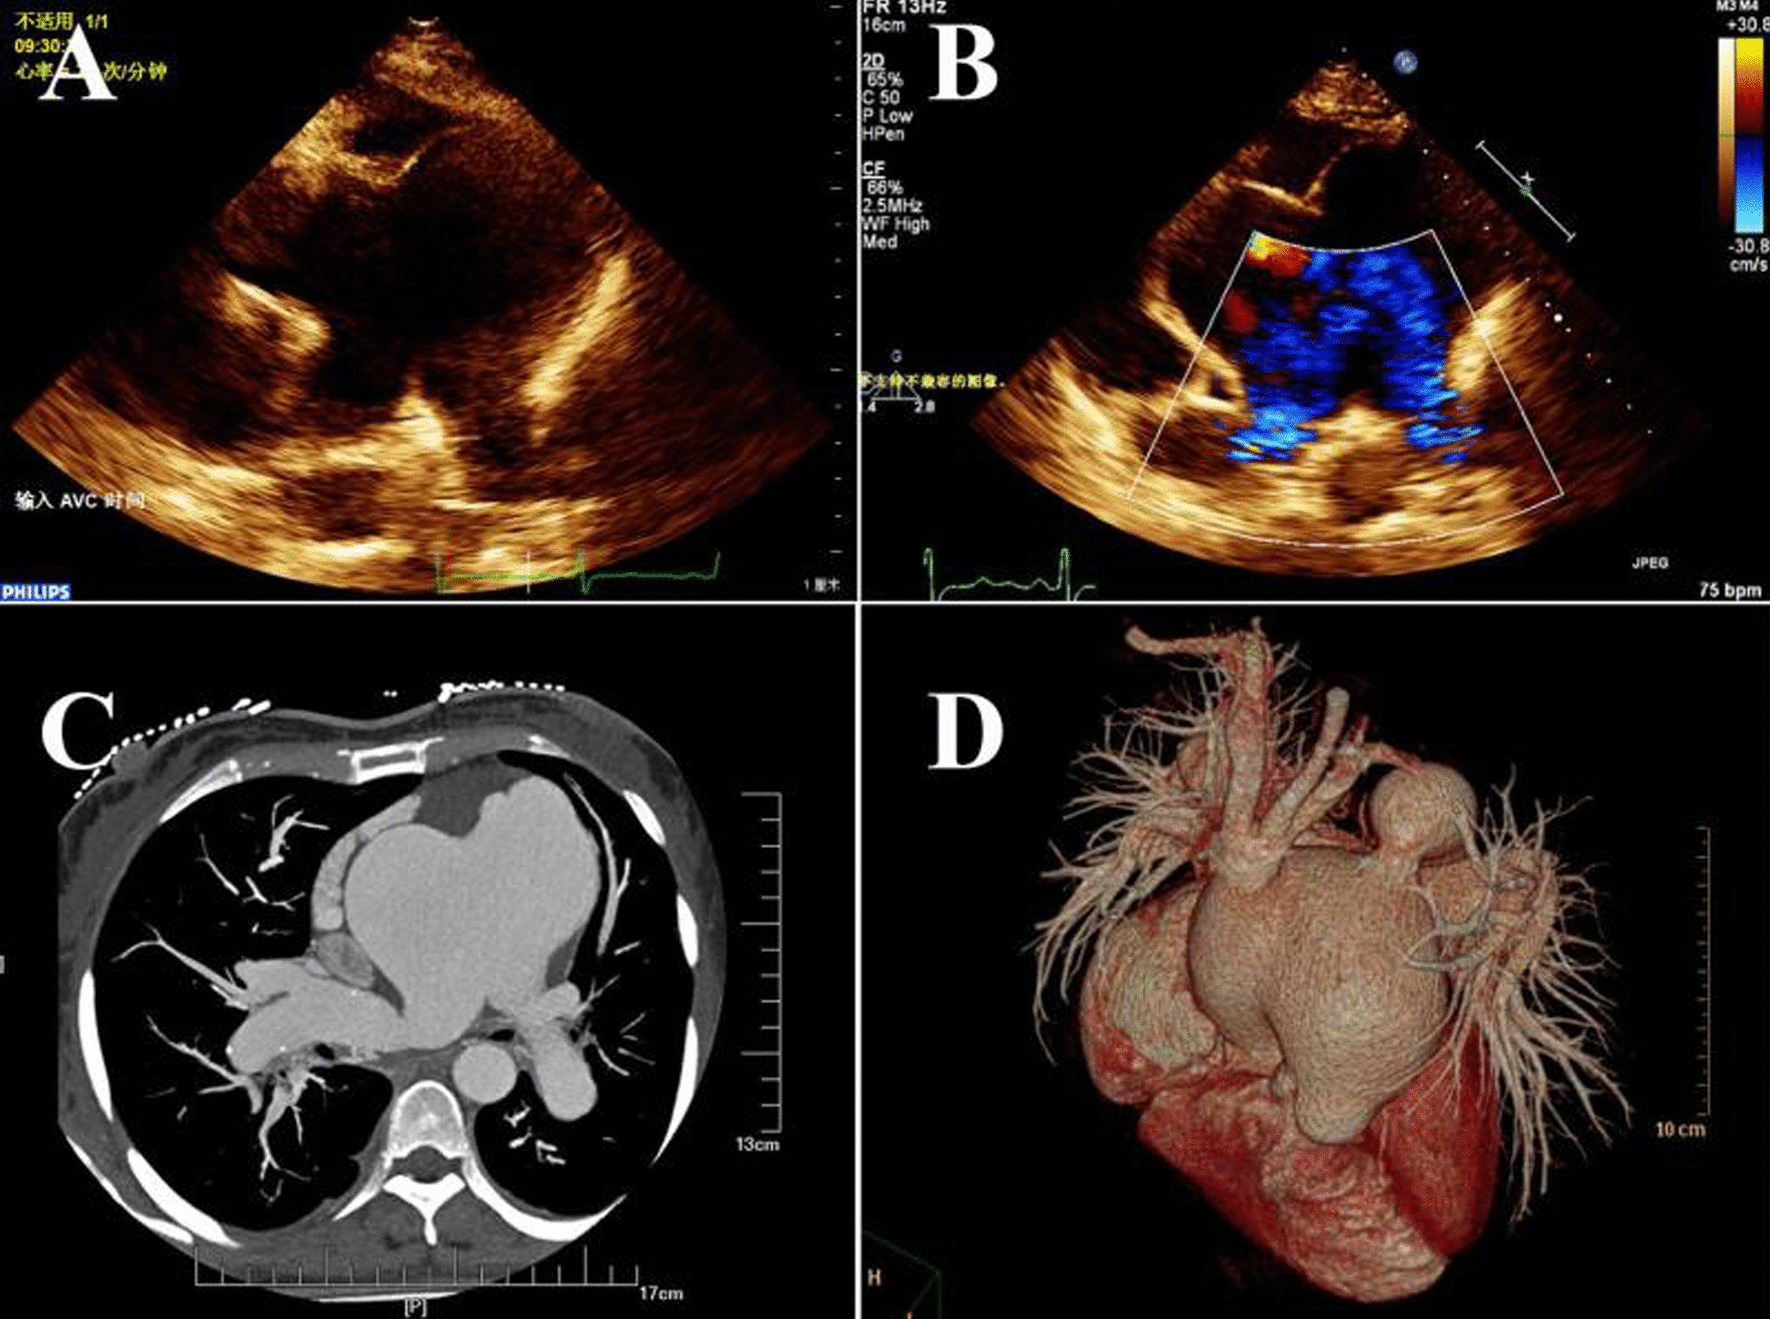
Transthoracic echocardiography demonstrated a severely dilated aorta and main pulmonary artery with a large intervening defect (a, b). Distal to the APW, the ascending aorta gave rise to the right pulmonary artery (a, b). Computed tomography showed a type III APW and an AORPA (c). Three-dimensional computed tomography revealed a dilated aortic sac, IAA, and the descending aorta formed via a PDA (d). AORPA, aortic origin of the right pulmonary artery; APW, aortopulmonary window; PDA, patent ductus arteriosus; IAA, interruption of the aortic arch
